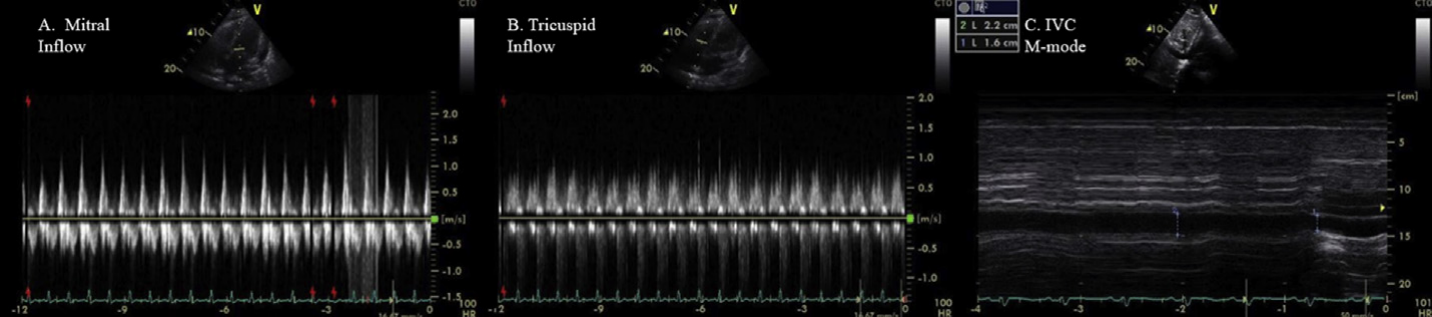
Pulsed-wave-Doppler recordings of (A) mitral and (B) tricuspid inflow showing mild respiratory variation of mitral and tricuspid inflows in setting of moderate pericardial effusion. (C) M-mode echocardiogram of IVC showing dilated IVC (>21 mm diameter) with plethora.
